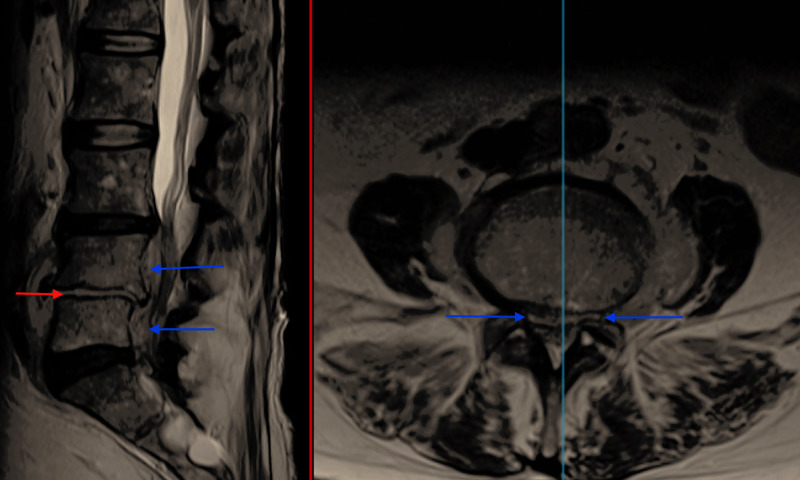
T2 TSE Sagittal and Axial MRI showing fluid in the L4/5 disc space (red arrow) and vertebral body oedema consistent with discitis and osteomyelitis along with an anterior epidural collection (blue arrows). TSE - Turbo Spin Echo; MRI - Magnetic Resonance Imaging
